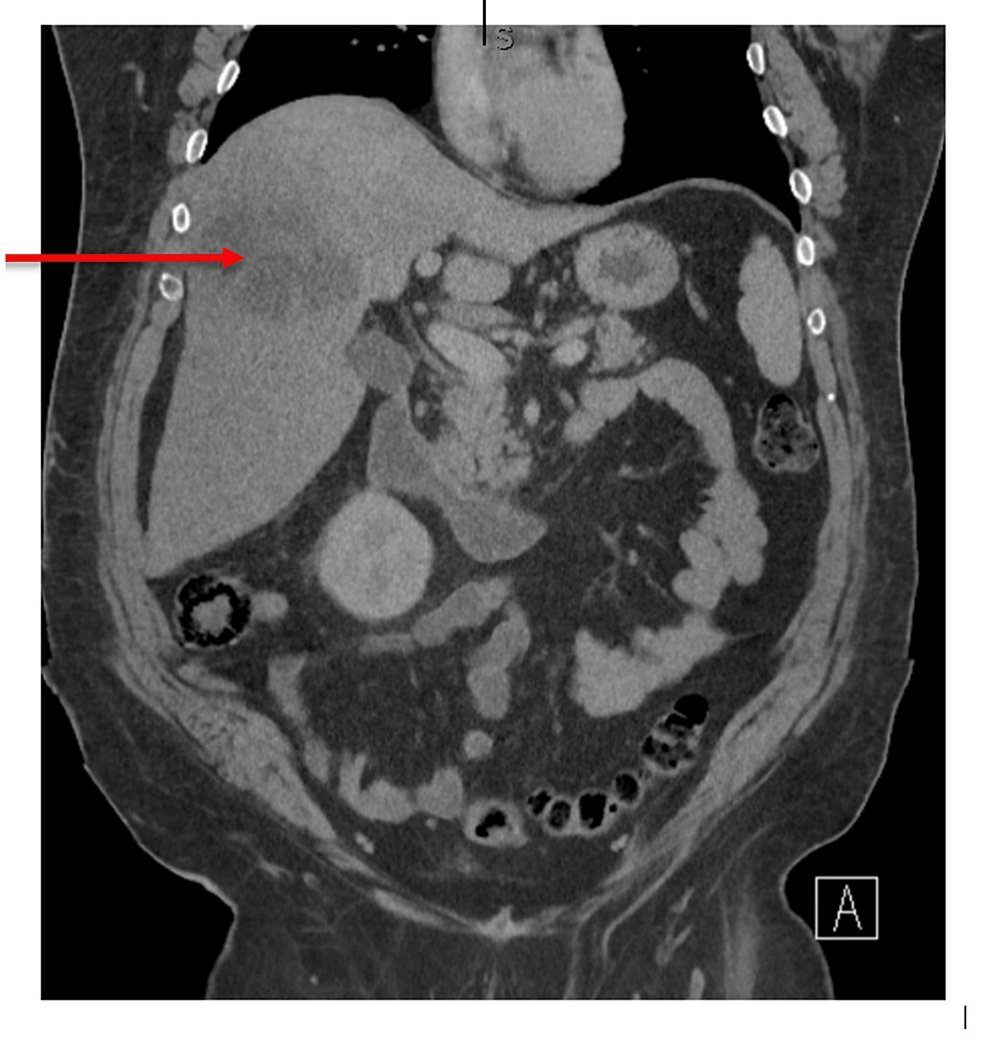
Computer tomography (CT) abdomen and pelvis without contrast findings: There is a large ill-defined hypoattenuating lesion or developing fluid collection within the right lobe of the liver, a lesion measuring collection within the right lobe of the liver, and a lesion measuring roughly 12.6 x 9.8 cm. The more central area of hypoattenuation measures slightly smaller, measuring up to 8.8 x 8.6 cm. There is decreased hepatic attenuation.
